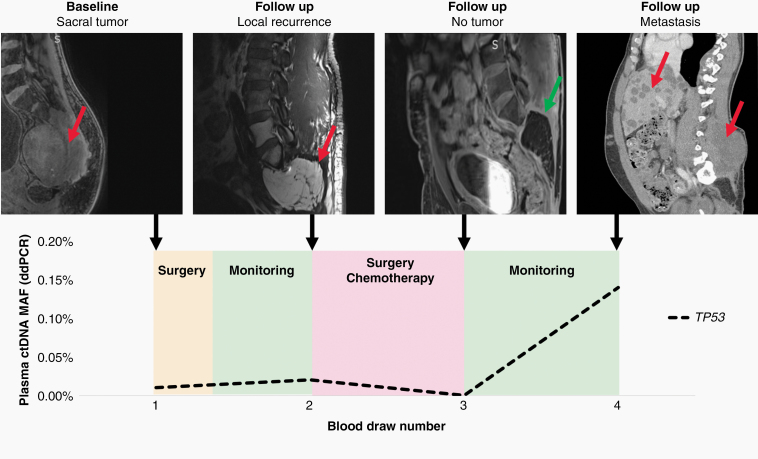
Liquid biopsy using ddPCR to detect mutations in *TP53* demonstrates concordance with radiographic monitoring during the follow-up period for patient 8134. At the time of diagnosis, ddPCR detected a mutation in *TP53* that was concordant with the whole exome sequencing data from the primary sacral tumor. Despite surgery, the patient presented with a locally recurrent tumor at the time of the second blood draw, which was reflected by a positive liquid biopsy result. After a second surgery, stereotactic radiotherapy, and chemotheray with imatinib and nivolumab, the patient's MRI was negative. When the patient returned for a final visit after being lost to follow-up for over a year, the patient had developed local recurrence and metasatic disease to the liver and lungs, which was detected by ddPCR. The red arrows identify tumor, while the green arrow shows no tumor recurrence at the primary site.
